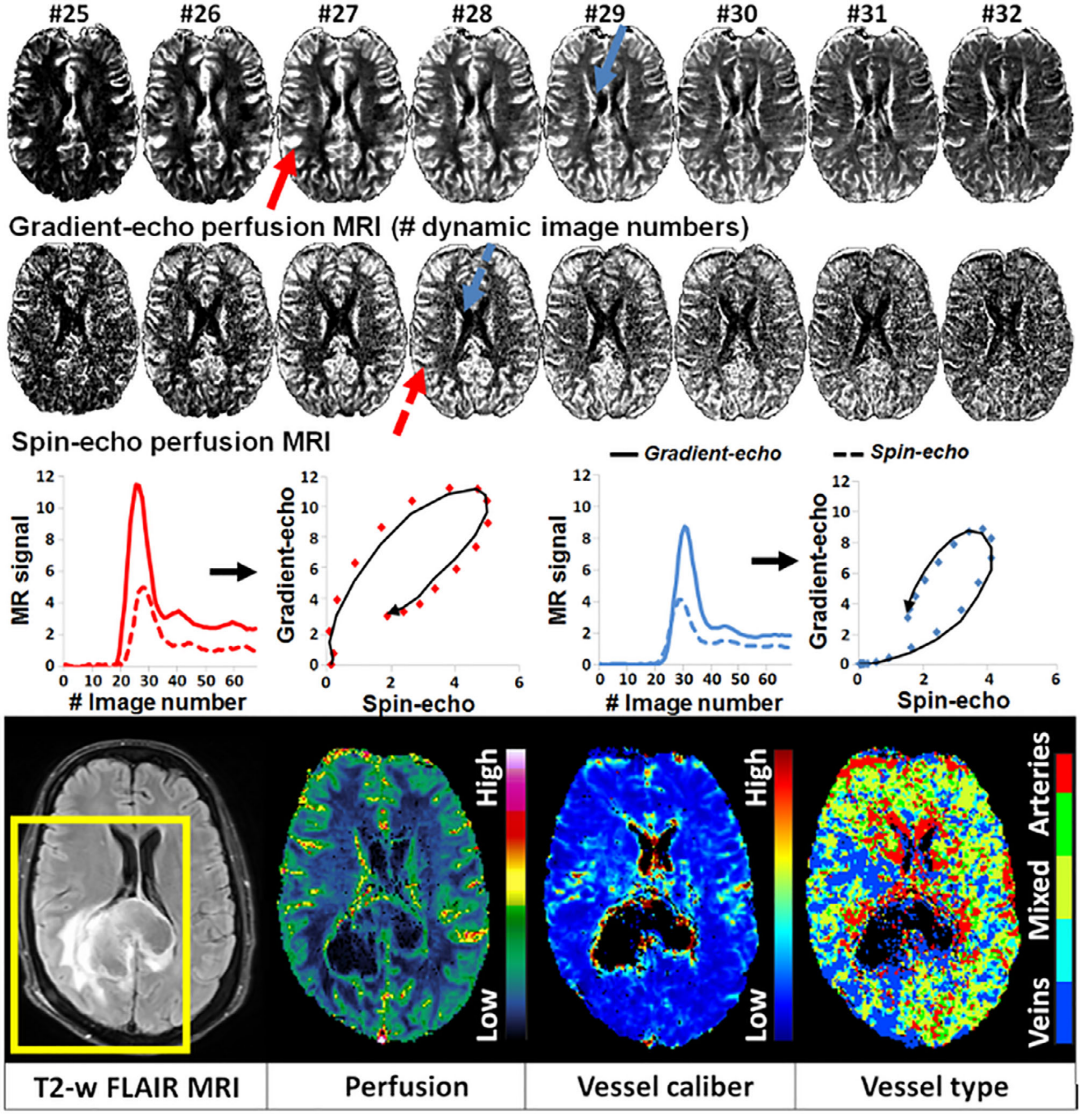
Perfusion MRI and vessel‐architectural imaging (VAI). Merging two perfusion readouts (gradient‐ and spin‐echo MRI) creates unique signal curve “loops” that scale with vessel calibers (slope) and vessel type (loop direction). Note the impaired, venous‐like dominance of the peri‐tumoral area (yellow box) on the vessel type map of a glioblastoma not observed on other images. Adapted from Emblem et al, Nature Medicine 2003.^92^
